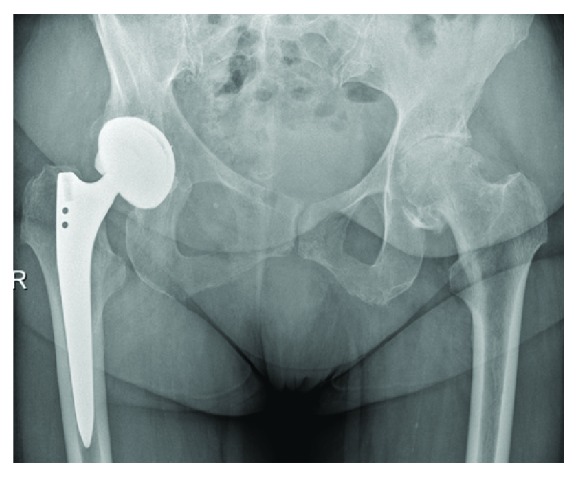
Preoperative X-ray showing the osteoarthritis of left hip.
